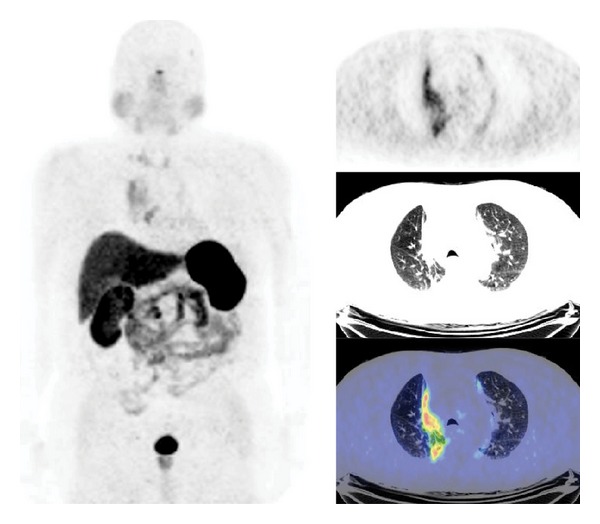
^68^Ga-DOTATATE PET/CT images (MIP, axial) in a case of metastatic atypical lung carcinoid.
